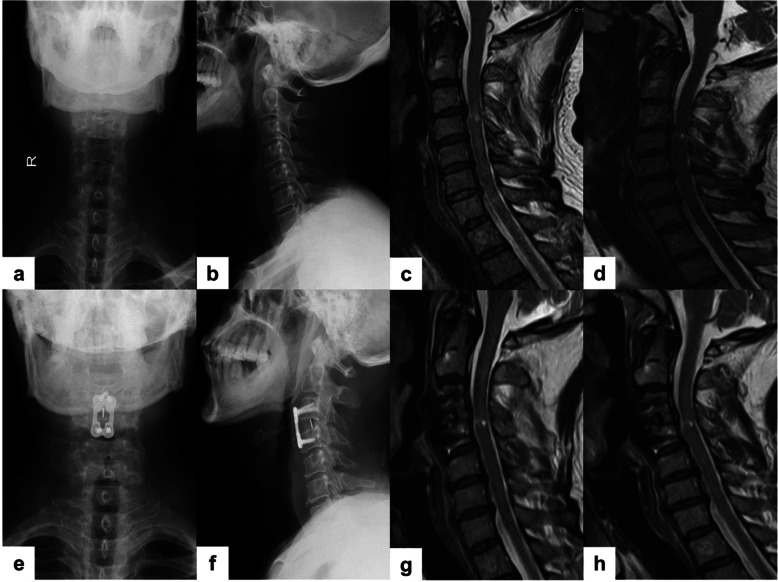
a, b X-ray showing no fracture and dislocation of the cervical spine. c MRI revealing long spinal cord high signal in acute phase. d Re-examined MRI showing that the high signal of the spinal cord was shorter than before. e, f Postoperative X-ray revealing that the internal fixation position was appropriate. g Postoperative MRI showing that the high signal of the spinal cord was shortened compared with preoperative. h Recent follow-up MRI revealing spinal cord hyperintensity limitation and signal intensity recedes
